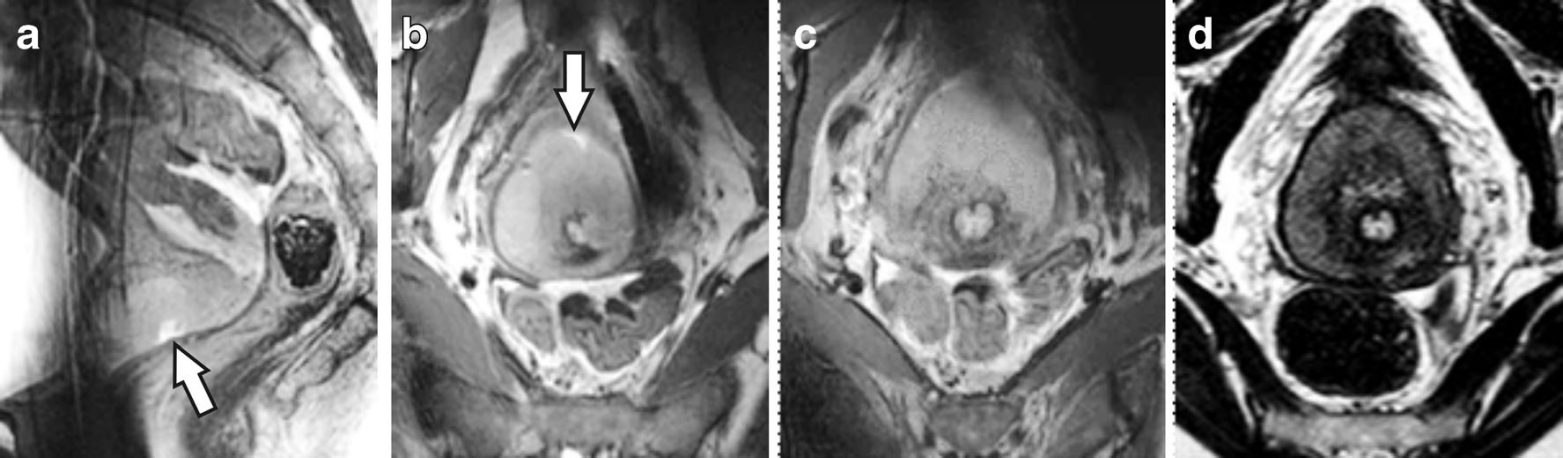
a Mid-sagittal and b axial oblique (perpendicular to the cervical canal) T_2_-weighted slice at 7.0 T of a 44-year-old patient diagnosed with a 70-mm, stage IB2, poorly differentiated squamous cell carcinoma originating from the ventral part of the cervix. Note the visible biopsy site (*arrow*). c Slice from the same sequence, though 12 mm cranially, as b, depicting part of the healthy (T_2_ hypointense) cervix invaded by tumour. d Axial oblique T_2_-weighted slice from the clinical 1.5-T MRI, created 17 days earlier, matched to c for comparison. Note the T_2_ hypointense fibrostromal ring surrounding the tumour
